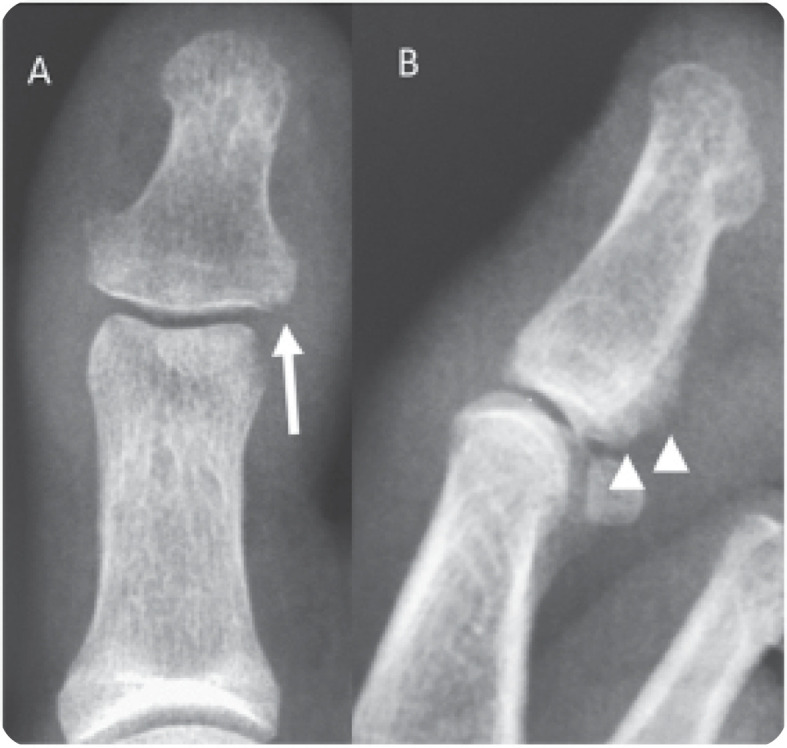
(right). Conventional radiography of the hallux with anteroposterior (A) and lateral (B) views showing periarticular erosions (arrows in A) and periosteal reaction (arrowheads in B) of the distal phalanx.
